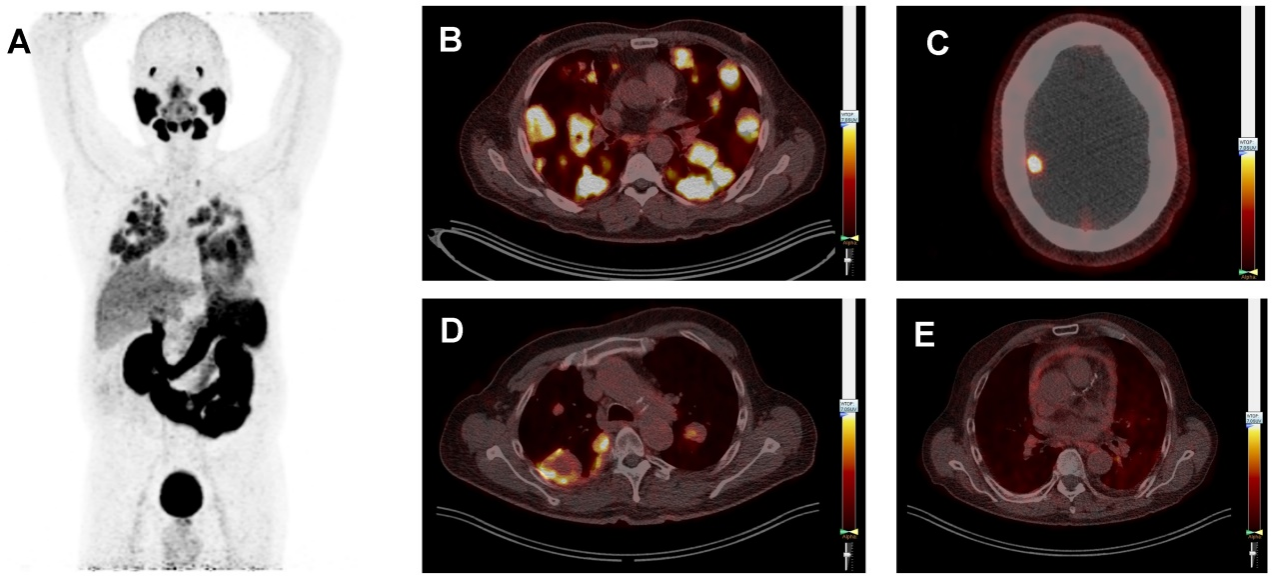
Typical prostate-specific membrane antigen (PSMA) positron-emission tomography (PET) whole body image of patient 3 (ACC) showing PSMA-ligand uptake in the lacrimal glands, salivary glands, lung metastases, liver, kidneys, spleen, intestine and bladder (A). Typical PSMA-PET/CT fusion images, all scaled at a SUV_max_ of 7.0, showing multiple lung metastases with homogeneous PSMA-ligand uptake in the same patient (B). Patient 21 (SDC) showing a brain metastasis (C). Patient 13 (ACC) showing lung metastases with inhomogeneous PSMA-ligand uptake and central photopenia. This patient was treated with cyclophosphamide plus doxorubicin plus cisplatin at the time of the PSMA-PET scan (D), and patient 25 (SDC) showing pericarditis carcinomatosa (E).
